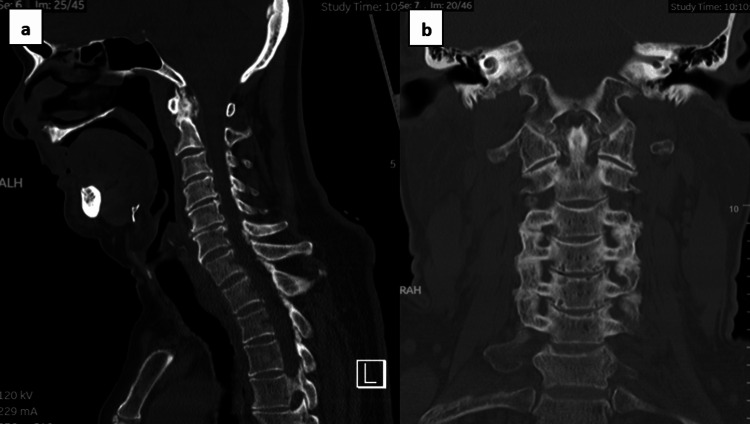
Computed tomography of the cervical spine. a) Sagittal view computed tomography of the cervical spine revealing osteophyte formation over the cervical vertebrae, with no evidence of ossification of the posterior longitudinal ligament. b) Coronal view computed tomography of the cervical spine showing uncovertebral hypertrophy without any observed vertebral fractures, subluxations, or dislocations.
